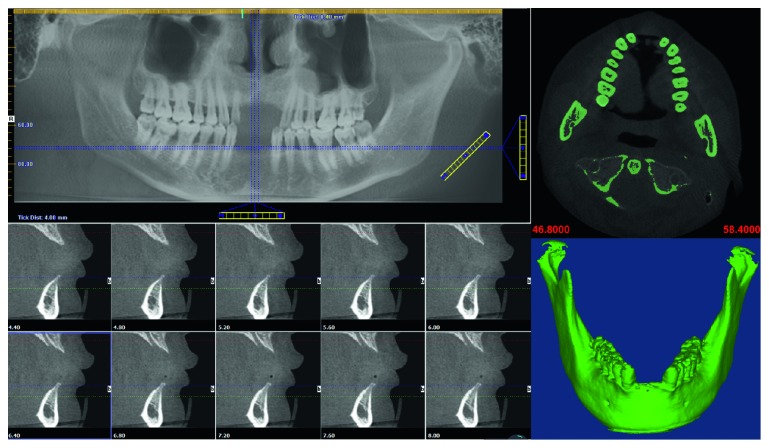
Preoperative CBCT scan and the 3D model of the patient's mandible.
